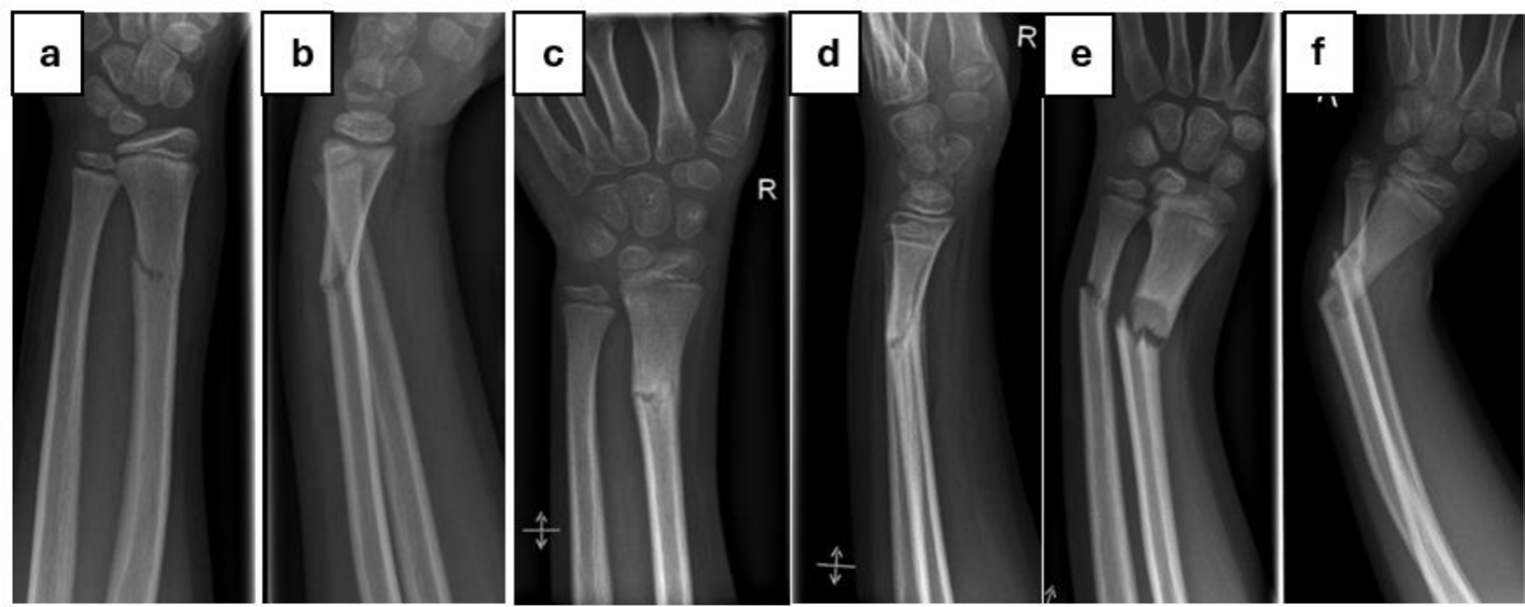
a – b, anterior-posterior and lateral radiographs of a diametaphyseal radius greenstick fracture in a 10-year-old boy at the day of the accident (day 0). The angle of the fracture line in the a.p. radiograph is approximately 25°. Hence, the risk for refracture according to the proposed risk prediction score in Table 4 is 29.5%; c – d, radiologic imaging shows consolidation in an acceptable position on day 33; e – f, radiologic imaging shows a refracture on day 147
